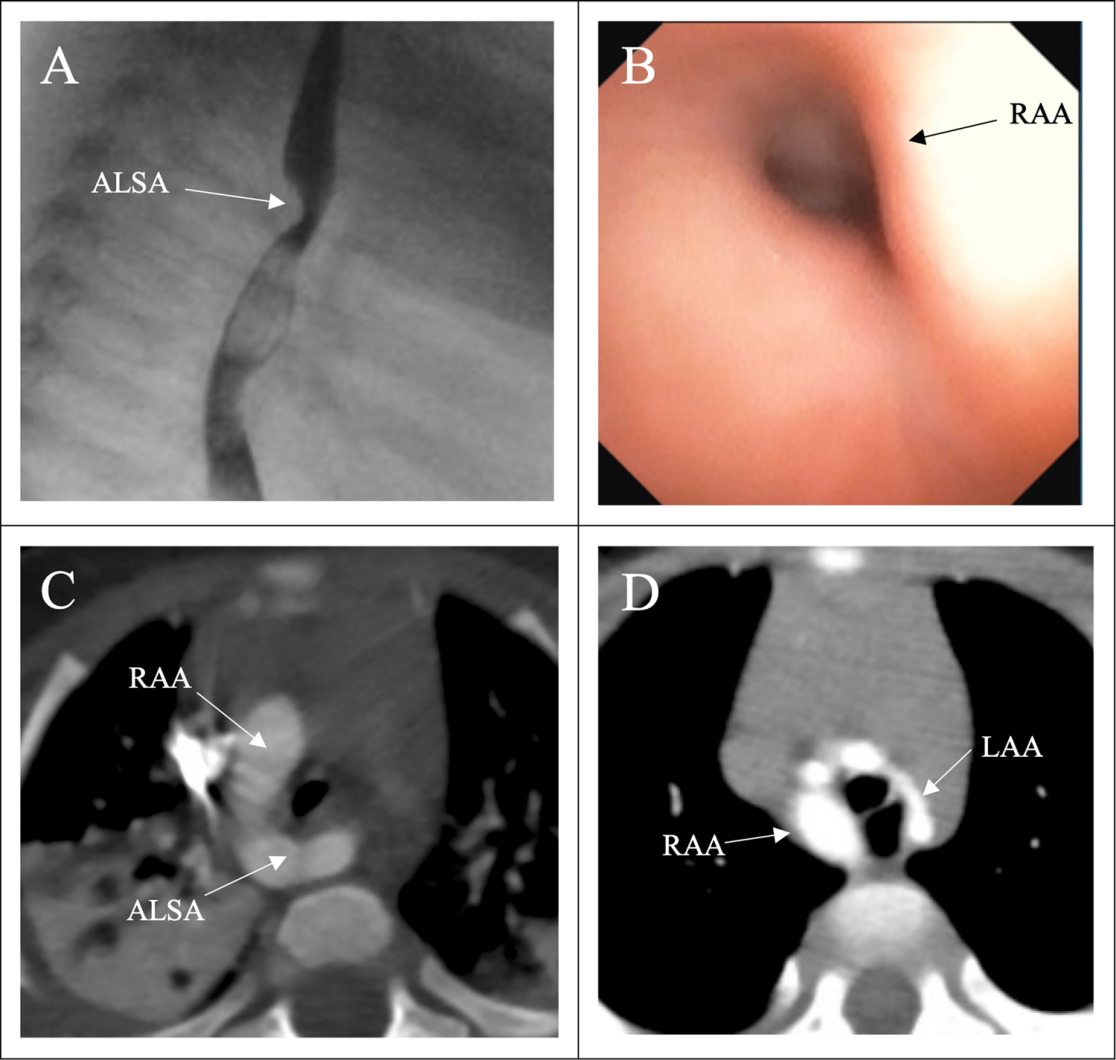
Symptomatic RAA arch evaluation. A. Esophagram demonstrating fixed posterior compression from aberrant left subclavian artery (ALSA). B. Distal tracheal compression by right aortic arch (RAA). C. Chest CT with right aortic arch and aberrant subclavian artery. D. Patient with prenatally diagnosed RAA, found to have right sided dominant double aortic arch and smaller atretic left aortic arch (LAA). [Color figure can be viewed at wileyonlinelibrary.com]
